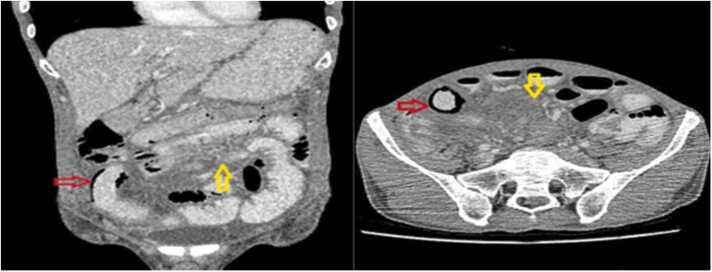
Axial and coronal contrast-enhanced abdominopelvic CT scan depicts increased density of ileal mesentery, representing an infiltrative process (yellow arrow). An ileal loop shows pneumatosis intestinalis in RLQ suggestive of transmural bowel necrosis (red arrow).
